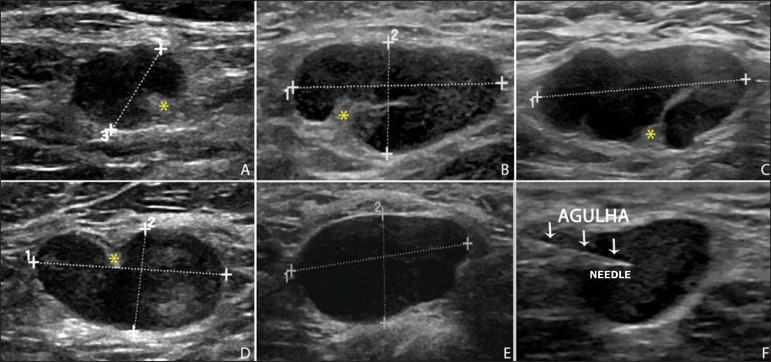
Sonographic images demonstrating some lymph nodes morphologically
